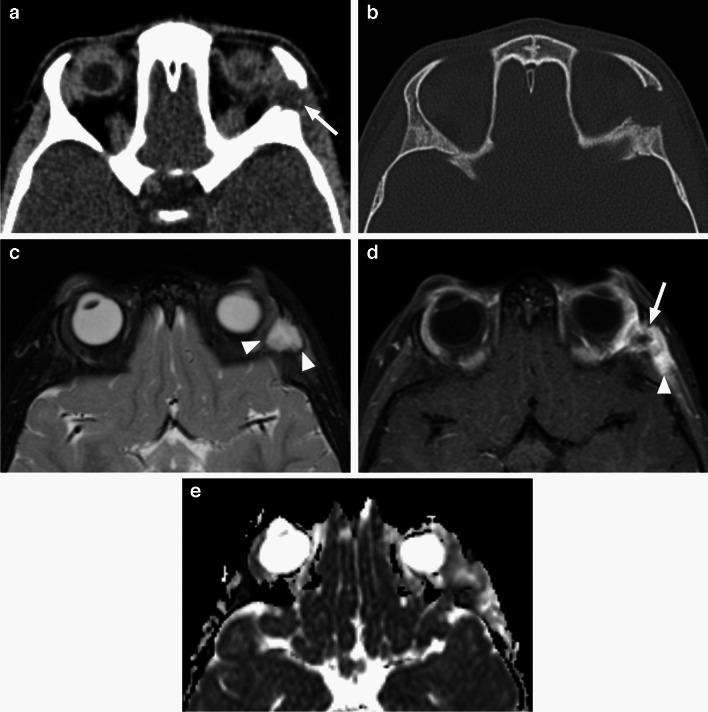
LCH. Axial nonenhanced CT image in soft tissue window and algorithm (a) shows a slightly hypodense osteolytic lesion (arrow) of the left superolateral orbital wall. Axial CT image at a similar level in bone window and algorithm (b) reveals smooth sharp borders of the destructed bone. Corresponding T2-weighted image (c) shows hyperintensity and a thin dark rim (arrowheads) of the lesion. There is predominantly peripheral enhancement (arrow), which extends into the surrounding tissues (arrowhead) on axial postcontrast T1-weighted image with fat saturation (d). The lesion shows centrally high and peripherally mild hyperintensity compared to the brain, consistent with high ADC values (increased diffusivity) on corresponding ADC map (e)
